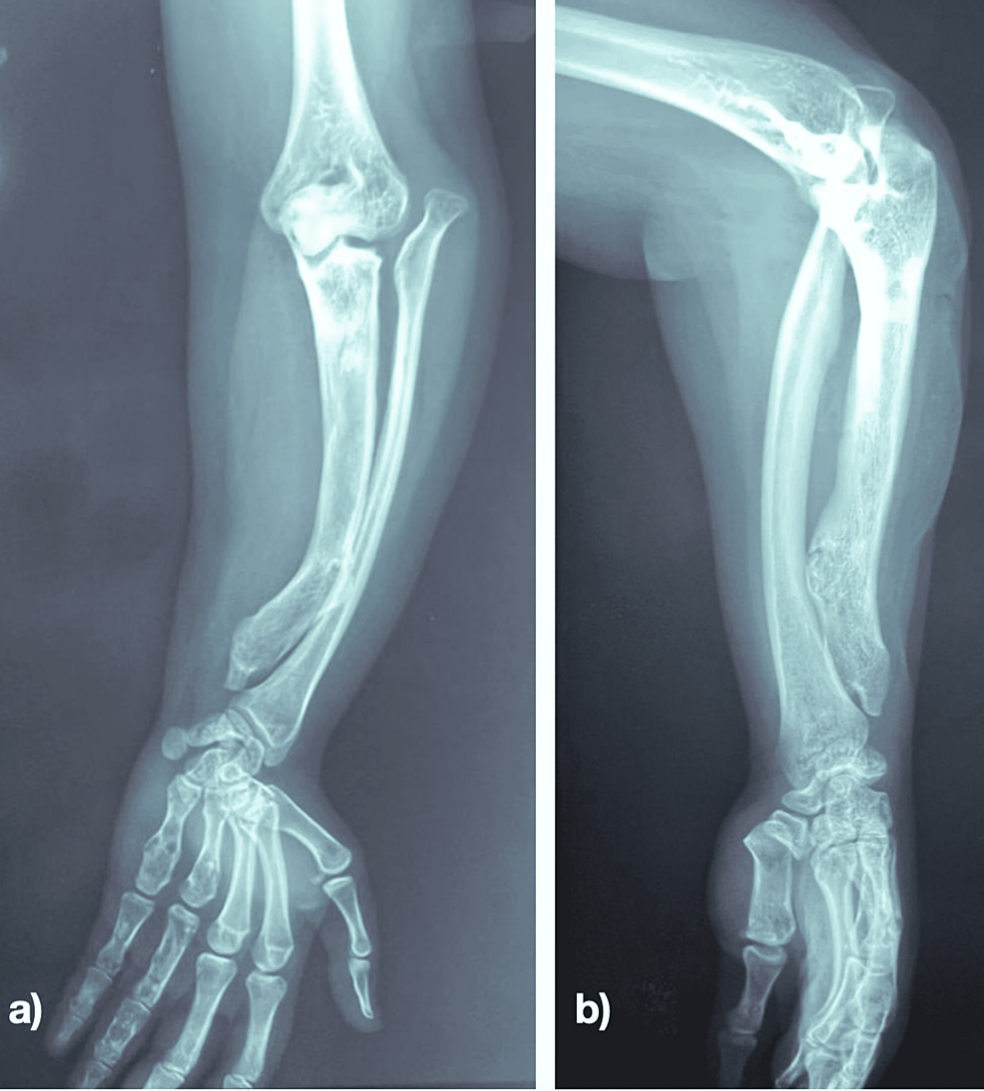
X-ray of the forearm before surgery: a) Anteroposterior X-ray of the forearm b) lateral X-ray of the forearm
